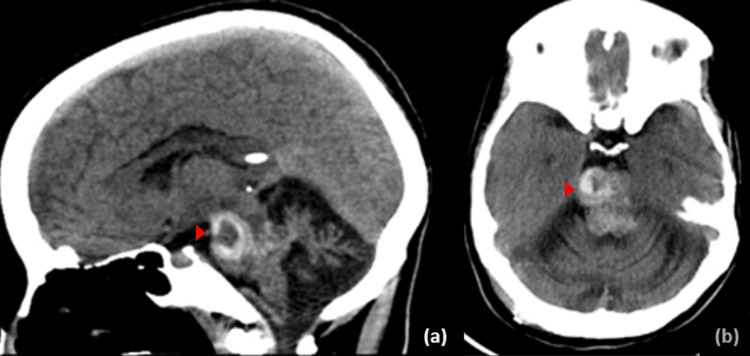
Urgent plain computed tomography imaging Sagittal (a) and axial (b) cuts of non-contrasted computed tomography scans showing an increase in the size of ventricles.
